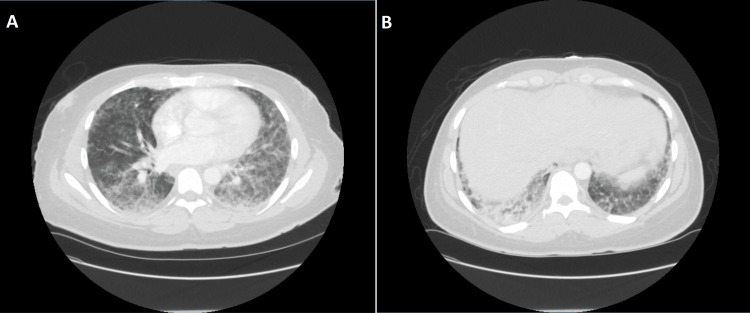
CTA chest A: Extensive diffuse bilateral ground-glass opacities; (B) Confluent region of consolidation in the right posterior lower lobe compatible with an infectious or inflammatory process CTA: Computed tomography angiography
